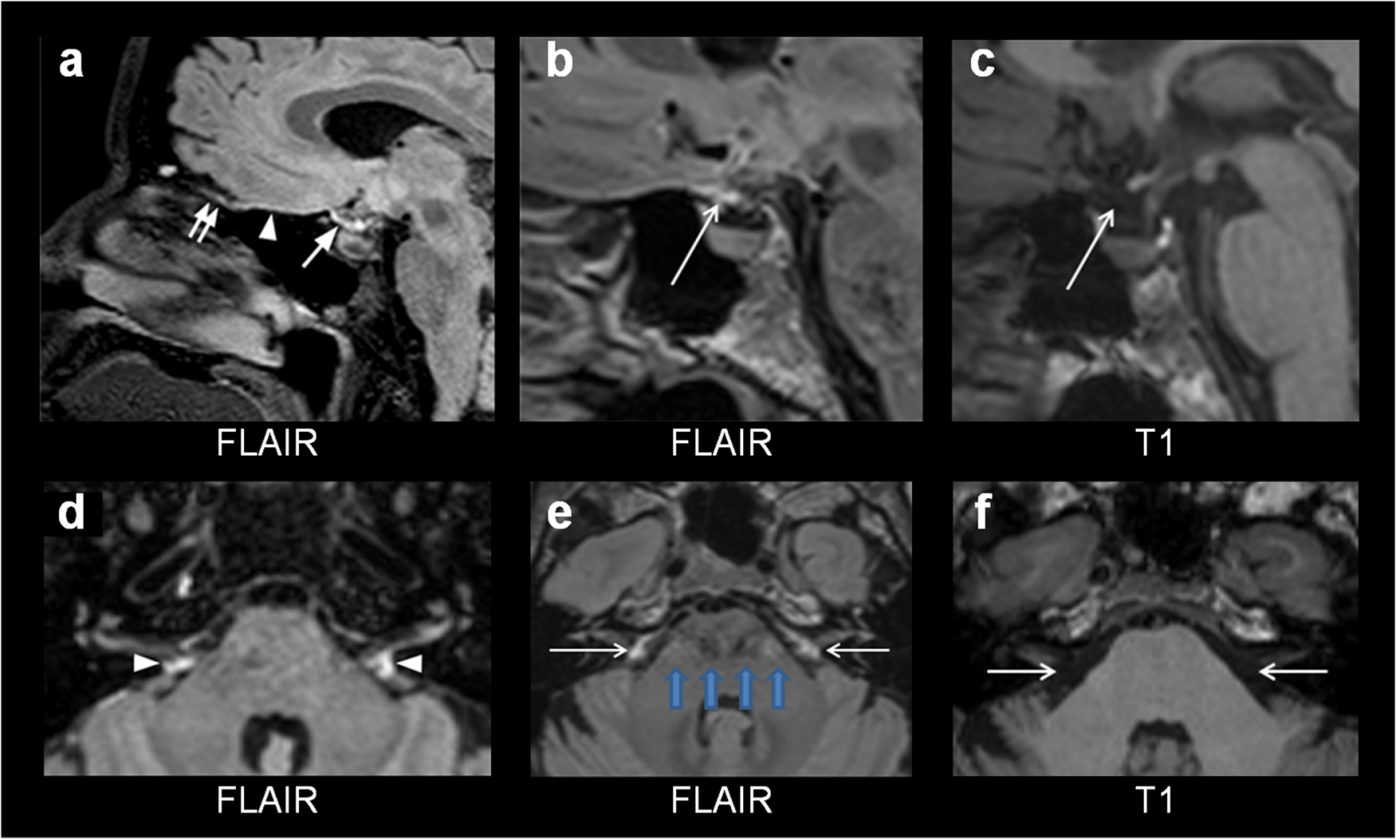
CSF flow artifacts at FLAIR in the subarachnoid space. a In their Figure 4a, Albayram et al.^3^ point at a linear hyperintensity at sagittal FLAIR in the suprasellar cistern and identify this as lymph. b Image from our same Prisma MRI scanner (Siemens, Erlangen) shows a similar phenomenon (arrow). c At T1 weighted MRI through the same section it becomes clear that the FLAIR hyperintensity is within the CSF compartment (arrows), not the dura itself, which in this region is constituted by the sellar diaphragm immediately adjacent to the upper border of the pituitary gland. This clearly indicates a flow artifact derived from CSF pulsations within the suprasellar cistern. d In their Figure 4f, Albayram et al.^3^ point at FLAIR hyperintensities (arrowheads) within the cerebellopontine angle cisternal spaces and identify them as lymph (axial section). e We reproduce similar findings (arrows) at our same MRI Prisma scanner (Siemens, Erlangen). Propagation of artifacts through the brain stem (blue arrows) in the same level (along the phase encoding direction), as also seen to a minor extent in d, is highly suggestive that these are CSF flow artifacts. f At T1 weighted MRI through the same imaging plane it becomes clear that the hyperintensities at FLAIR correspond to the CSF space (arrows), not the dura or cranial nerves.
